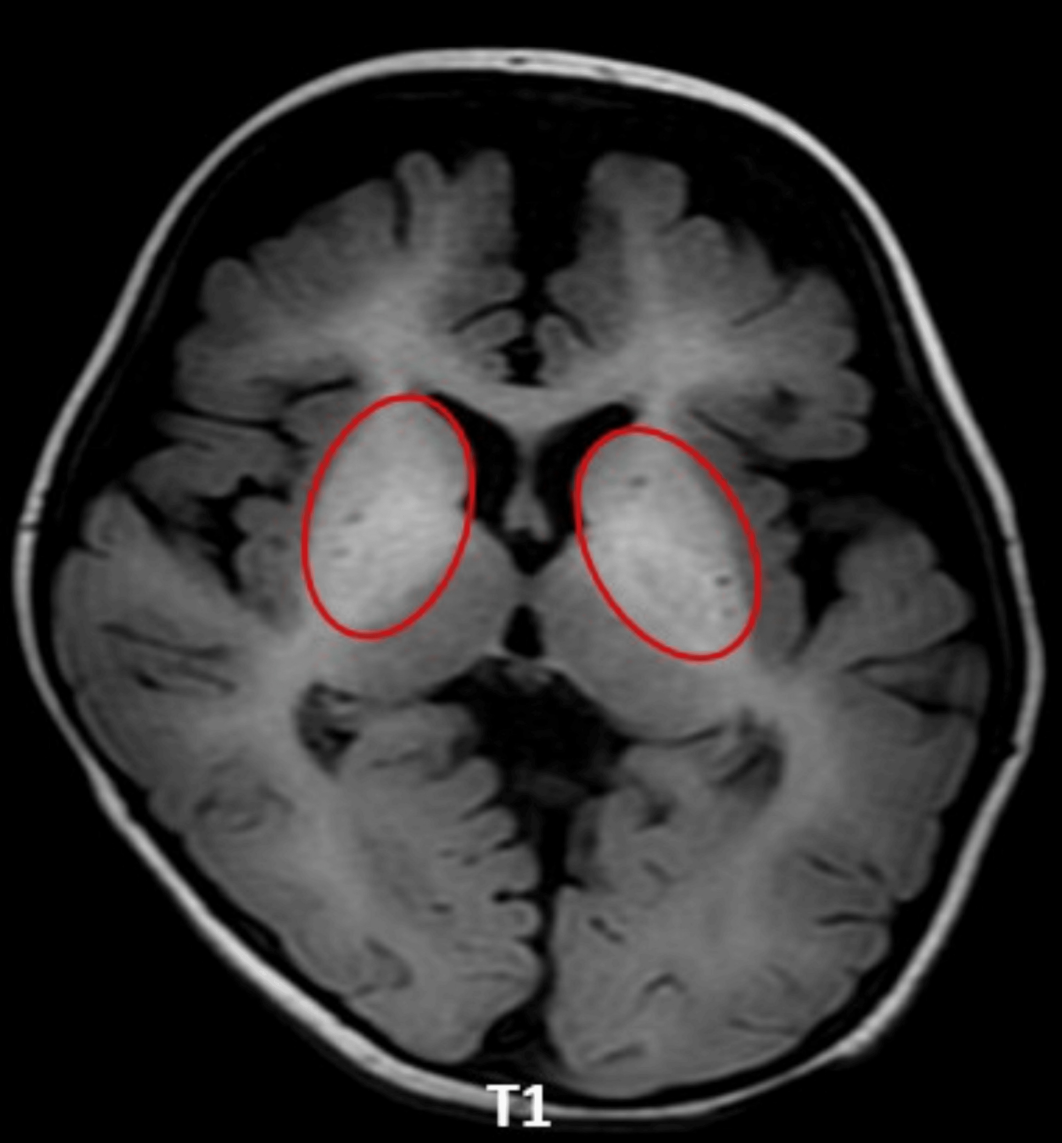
Axial T1 sequence of MRI brain shows hyperintensity of the white matter (red circles). MRI: magnetic resonance imaging
